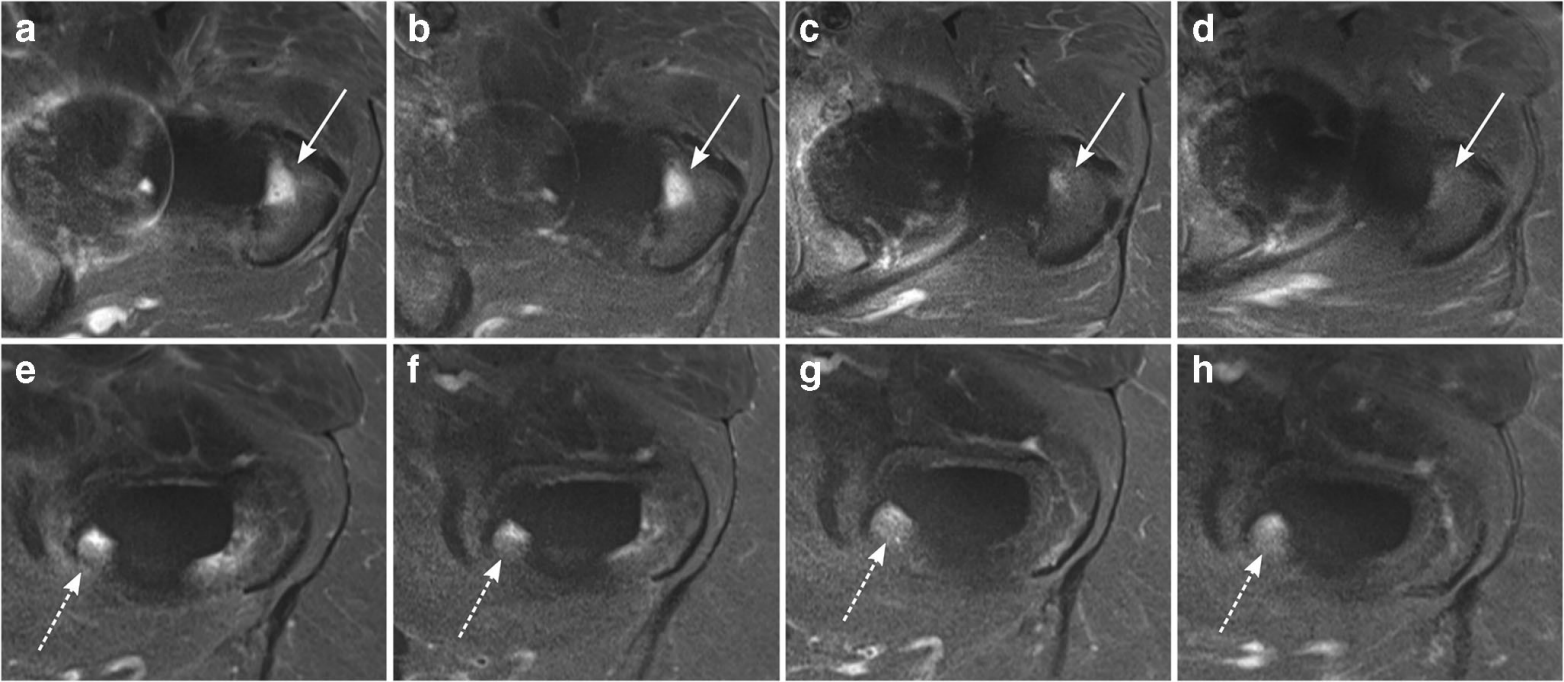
BME around the femoral stem on axial STIR MRI. A 68-year-old asymptomatic woman with left-sided THA and BME pattern around the femoral stem in Gruen zone 1 (arrows) decreasing over time (a = 3 months, b = 6 months, c = 12 months, and d = 24 months after THA). Persisting BME in the same patient in Gruen zone 7 (dashed arrows; e = 3 months, f = 6 months, g = 12 months, and h = 24 months after THA). *BME*, bone marrow edema; *THA*, total hip arthroplasty
